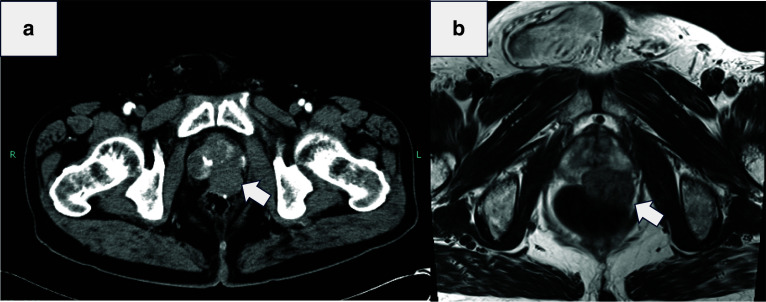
CT and MRI findings. a CT showed a homogeneously contrast mass on the anterior rectal wall. No distant metastasis or enlarged lymph nodes were observed. b T2 weighted MRI demonstrated that the tumor was compressing the prostate, but there was a clear border between the prostate and the tumor
